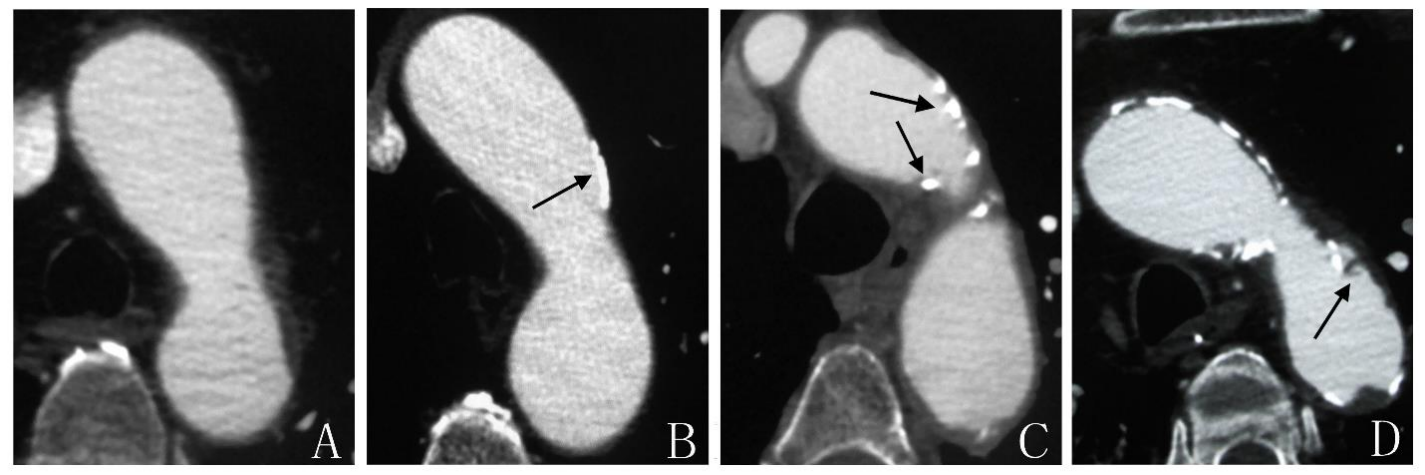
Axial CTA images of aortic plaques in four grades. A) No plaque at the aortic arch. B) a calcified plaque at the lateral wall of aortic arch with thickness less than 4 mm. C) Multiple mixed plaques at the aortic arch and descending aorta which are thicker than 4 mm. D) ulcer plaque at the lateral wall of aortic arch (black arrows indicate plaques).
